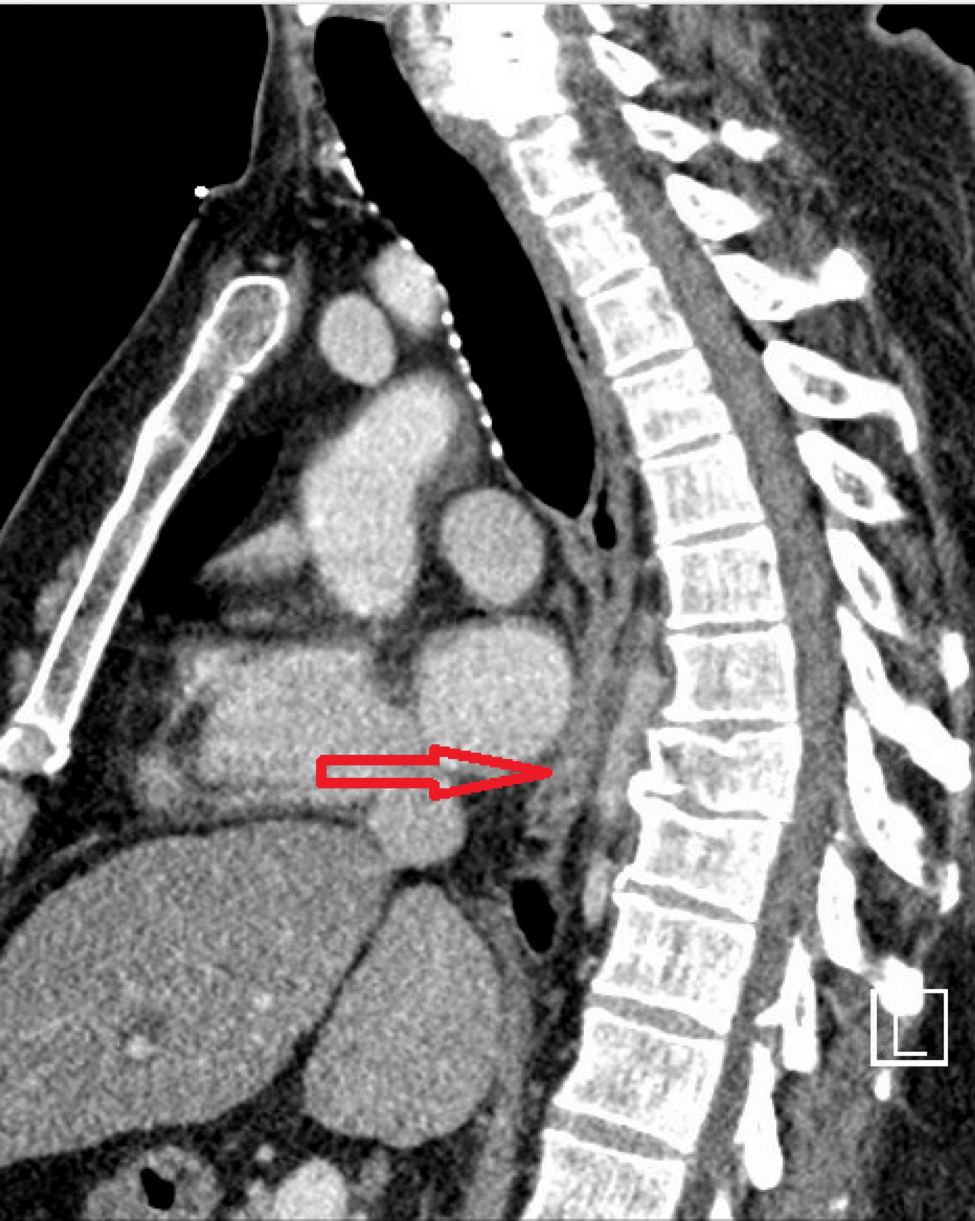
CT imaging of T8 fracture on the day of fracture.
